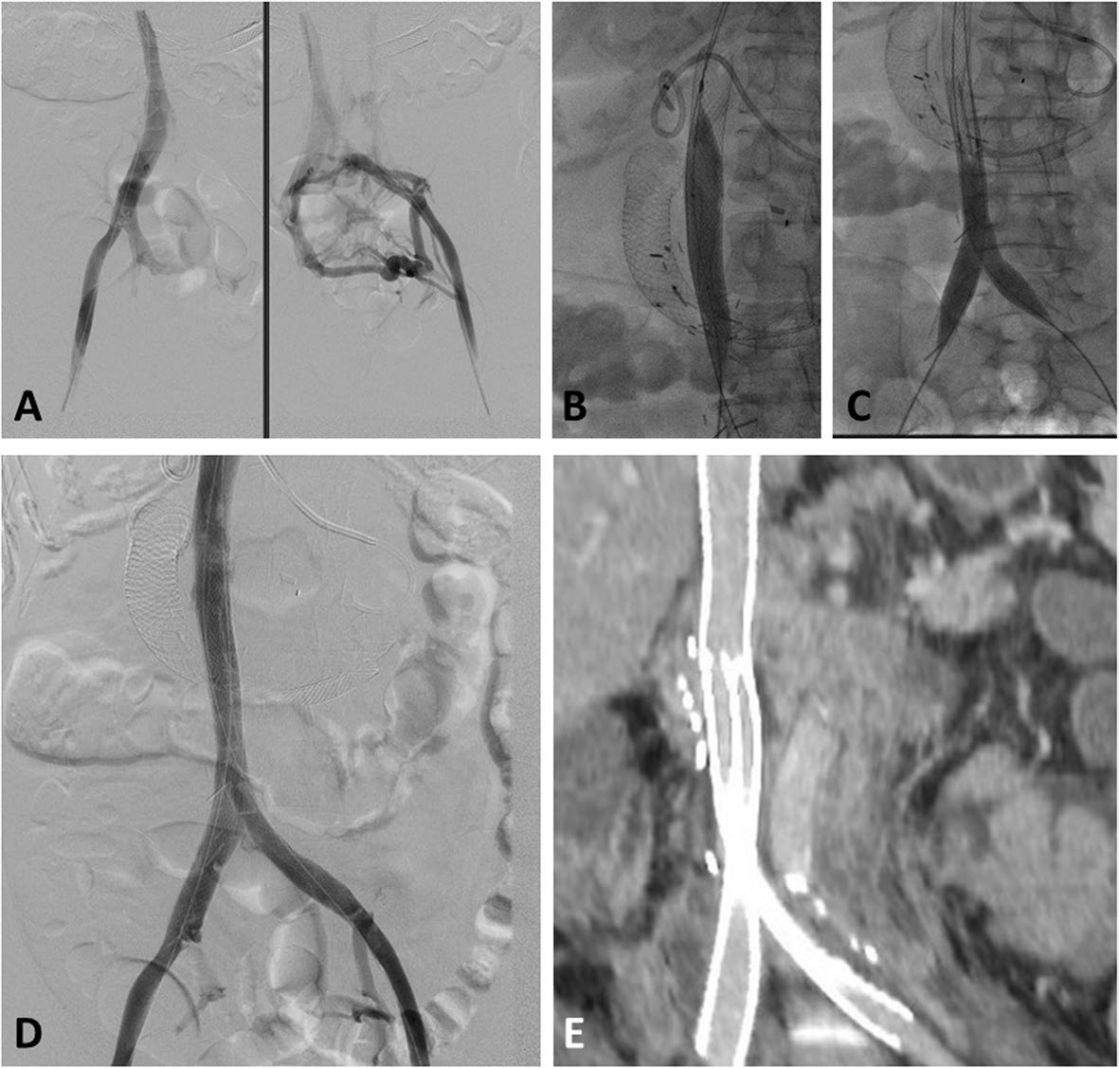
60 Y Female with RCC presenting with new lower extremity swelling due to Iliocaval compression by retroperitoneal masses A Initial venogram images obtained by bilateral femoral vein injection showing iliocaval obstruction with multiple collaterals B 24 mm Wall Stent deployed in the IVC with post stenting dilatation with 22 mm balloon. C bilateral kissing 18mm Iliocaval Wall stents deployed followed by stent dilatation with 16 mm balloon. D Final venogram showing restoration of in-line flow. E) Curved reconstruction of coronal follow up CT image showing preserved stent patency
